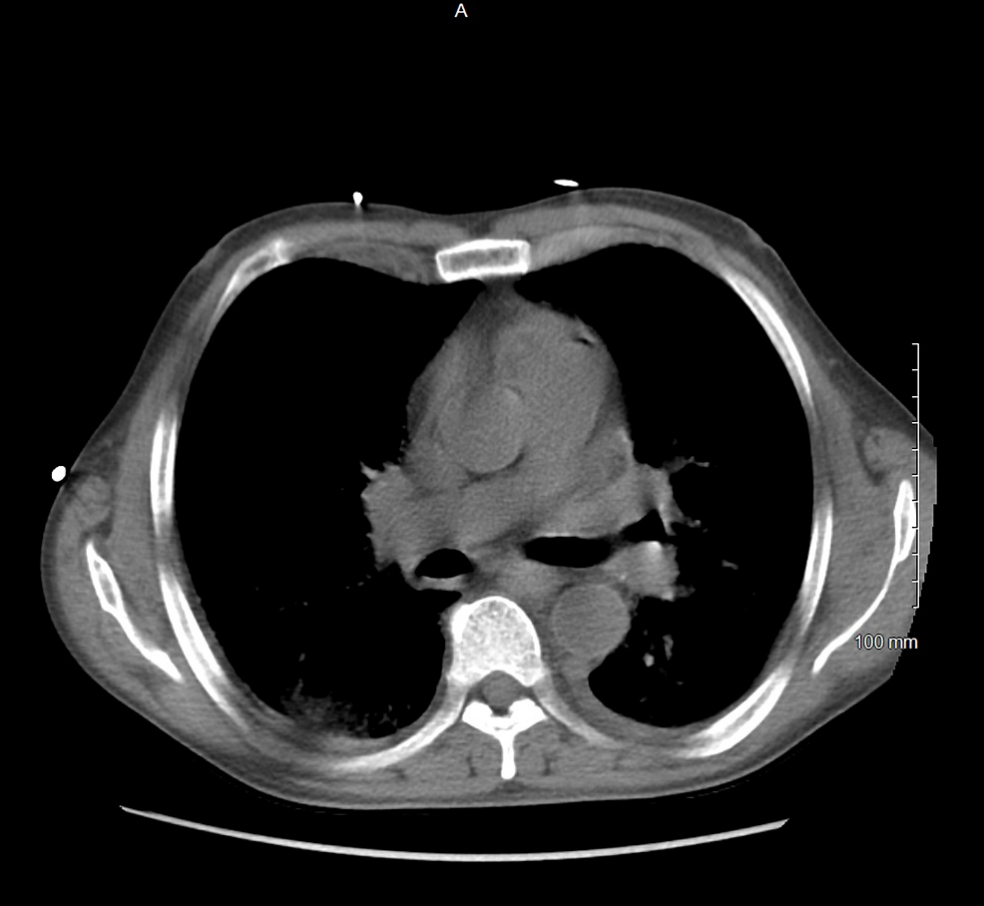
CT scan of the chest (sub carina level, mediastinal window) in the patient with acute histoplasmosis and HIV with no evidence of lymph node enlargement.
